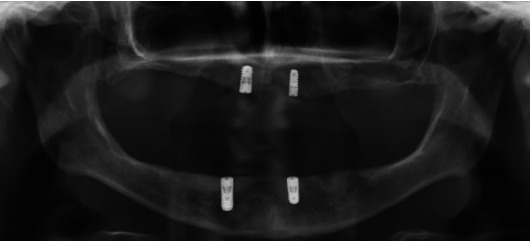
Panoramic radiograph (OPG) after implant placement and exposure at the time of impression taking. The correct fit of the scan bodies was ensured with an X-ray.
